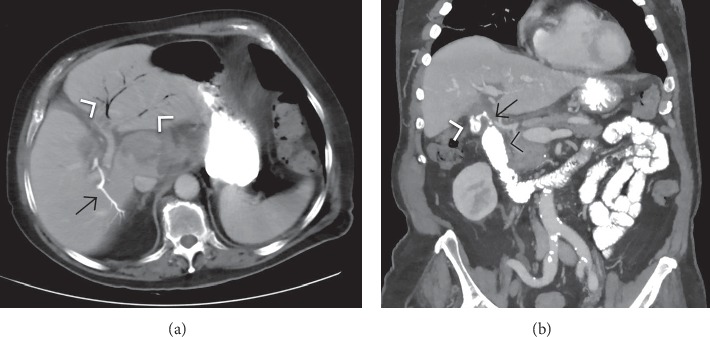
Contrast-enhanced computed tomography, 5-mm thickness multiplanar reconstruction, portal phase, demonstrates in (a) both pneumobilia (arrowheads) and opacification of the right lobe biliary branches (arrow) after oral contrast media administration; the fistula (arrow) between the gallbladder filled with contrast material (white arrowhead) and the duodenum (black arrowhead) is delineated in the coronal plane reconstruction shown in (b).
